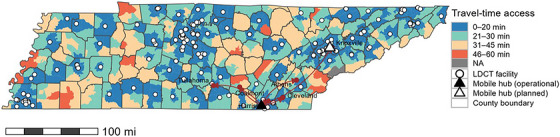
LDCT access bands and the distribution of fixed and mobile facilities. LDCT, low‐dose computed tomography.
